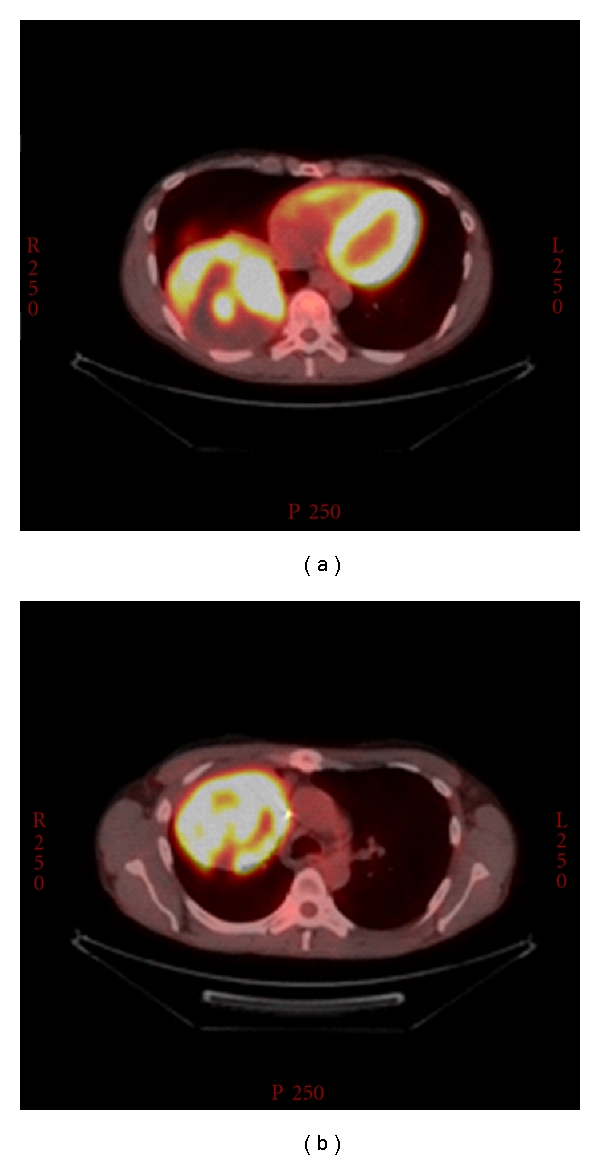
18F-FDG coincidence scintigraphy showed increased FDG uptake in right lung masses.
